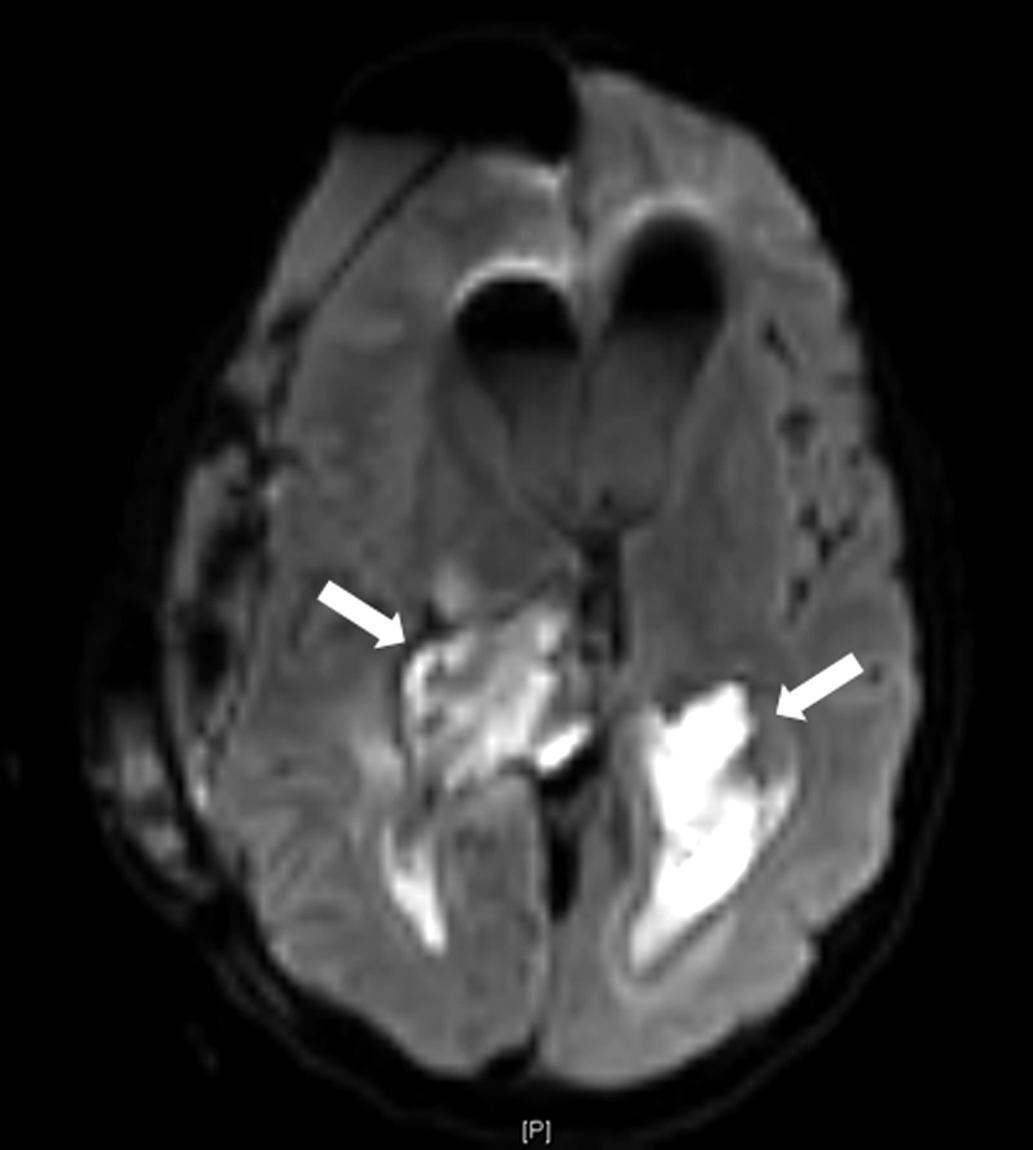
DWI MRI Note the diffuse intraventricular restriction on both lateral ventricles (arrows), characteristic of subdural empyema and severe ventriculitis. DWI: diffusion-weighted imaging; MRI: magnetic resonance imaging
